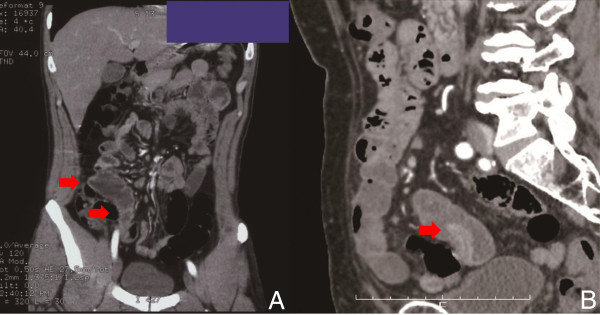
CT findings of Meckel’s diverticulum. A: Abdominal CT showed an extended part in the distal ileum. Continuity between the extended part and small intestine was unknown (Case 7). B: Abdominal CT revealed a polypoid lesion in the distal ileum. The peripheral branch of the ileocolic artery entered Meckel’s diverticulum. The polypoid lesion was regarded as Meckel’s diverticulum (Case 10).
